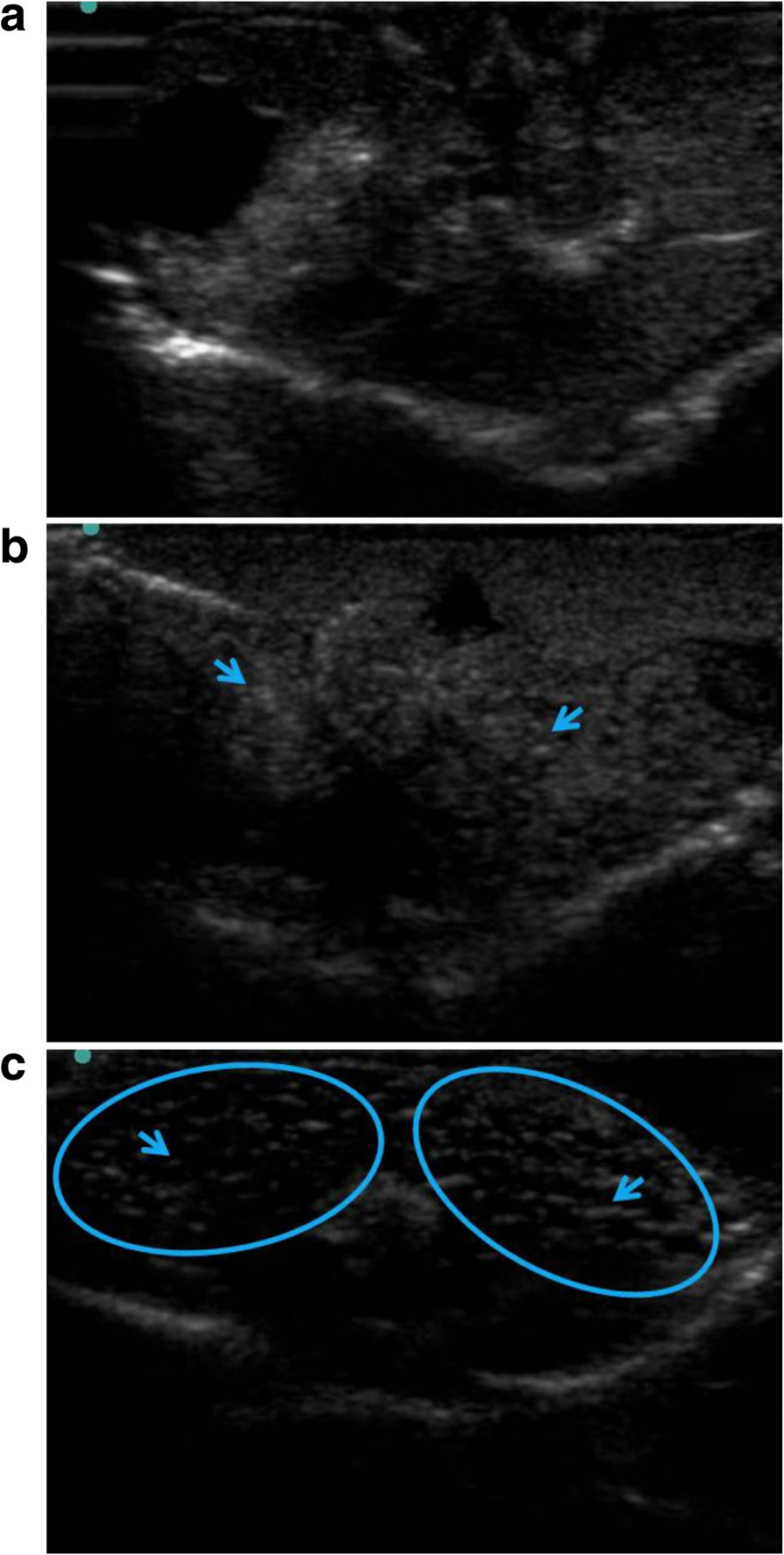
Ultrasonography images used for DGF sex identification. Panel a depicts a trans-abdominal ultrasound scan of a male, while Panels b and c show female scans with low and high follicular development, respectively. Arrows indicate examples of follicles. Large patches of well-developed follicles are circled (panel c) to highlight the characteristic spacing and pattern of light and dark areas found in the abdomen of a gravid female. Females with low follicular development generally have smaller follicles and show less space between them (panel b)
